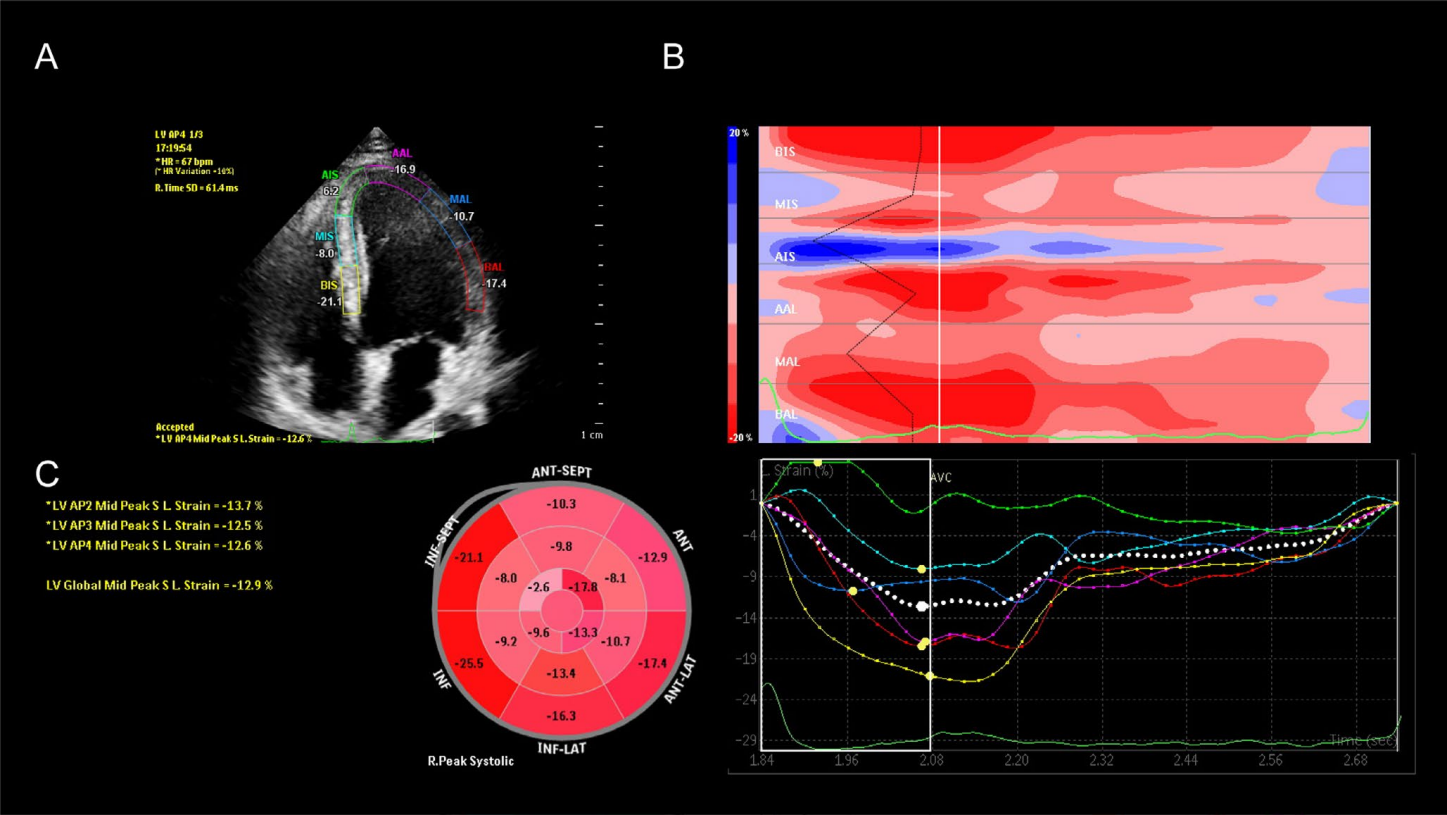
LS-STE and CMR-FT analysis. Notes: A: Example of a patient the apical four chamber view, measurement of LS-STE. B-C: Example of a patient the long axis section, measurement of CMR-FT
